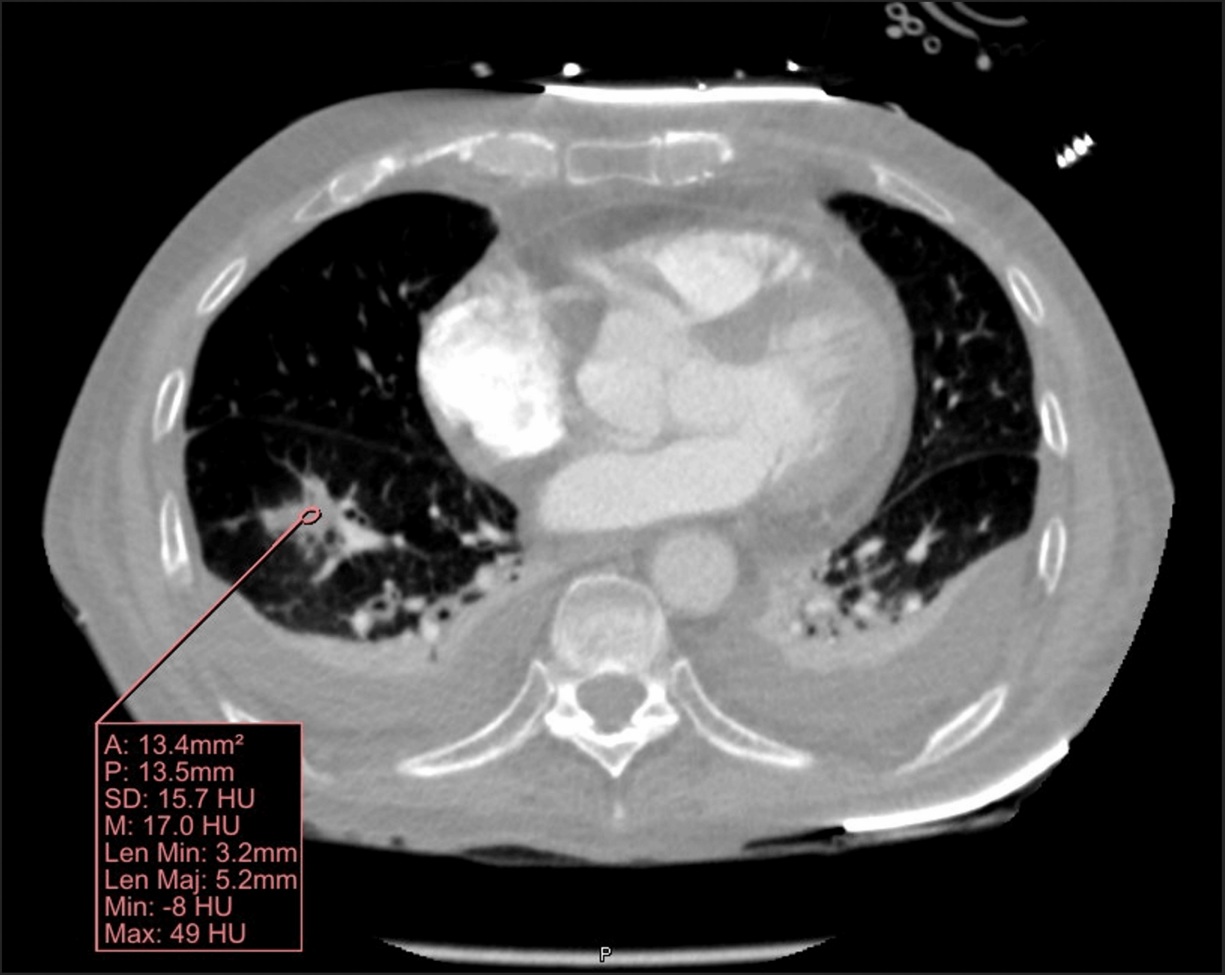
CT angiogram demonstrating a new septic pulmonary embolism.
